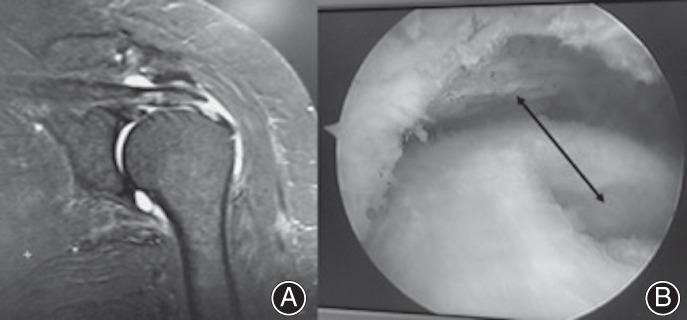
MRI images and findings during surgery. (A) Preoperative MRI images. (B) The corresponding rotator cuff injury in arthroscopy. The arrow showed the correspondence between injury and osseous proliferative structure of acromion.
